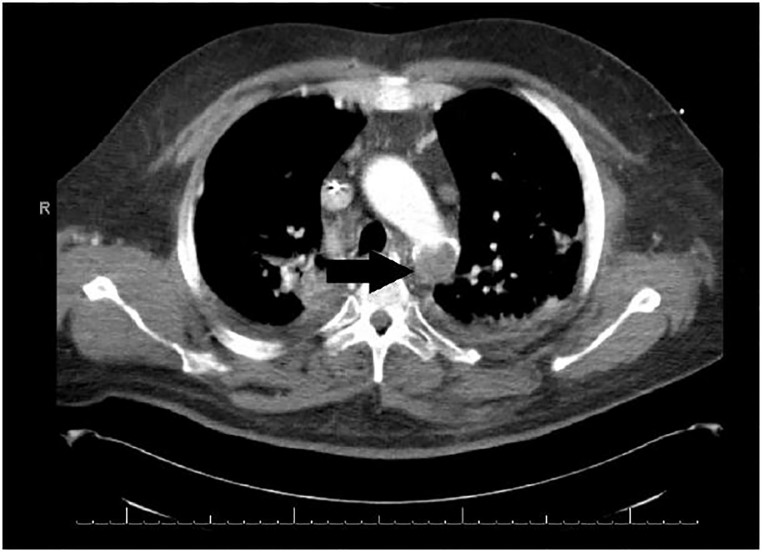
Computed tomographic angiography in axial view demonstrates complete occlusion of the proximal descending thoracic aortic stent (arrow).
